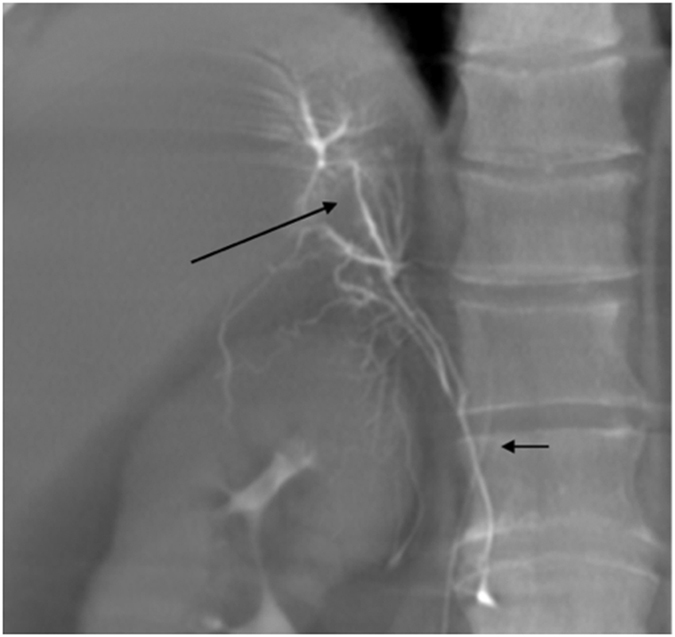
A 48-year-old male with primary hyperaldosteronism. The accessory hepatic vein (long arrow) was opacified during Dyna CT with the catheter tip in the right adrenal vein. The communicating capsular vein of the right adrenal gland was also shown (short arrow).
